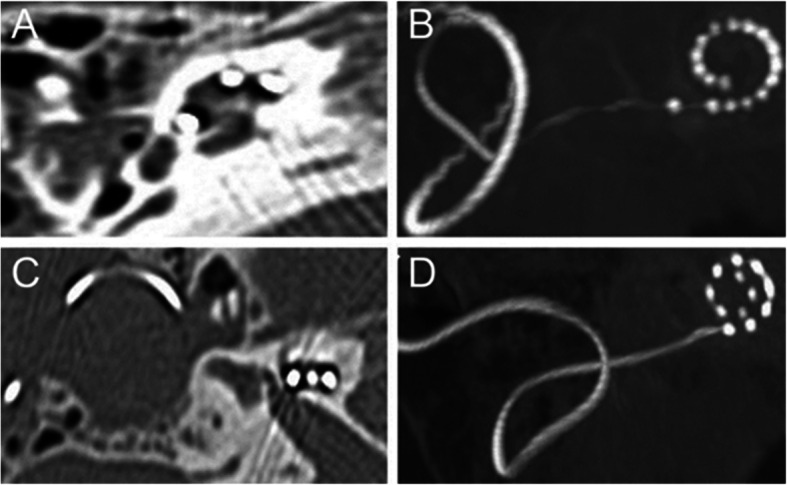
CT image of cochlea after using SEMAR combined with MIP. Counting electrodes using the SEMAR CT image requires superimposition of electrodes from different layers to count the electrodes in the cochlea, which requires complex operations (a, c). Using SEMAR combined with MIP enables us to directly count the cochlear electrodes in a single image. Advanced Bionics cochlear implantation: excluding the reference electrode (1) outside the cochlea, the number of electrodes inside the cochlea was 16 (b). Combi 40+ MED-EL cochlea implantation: 12 electrodes were counted (d)
